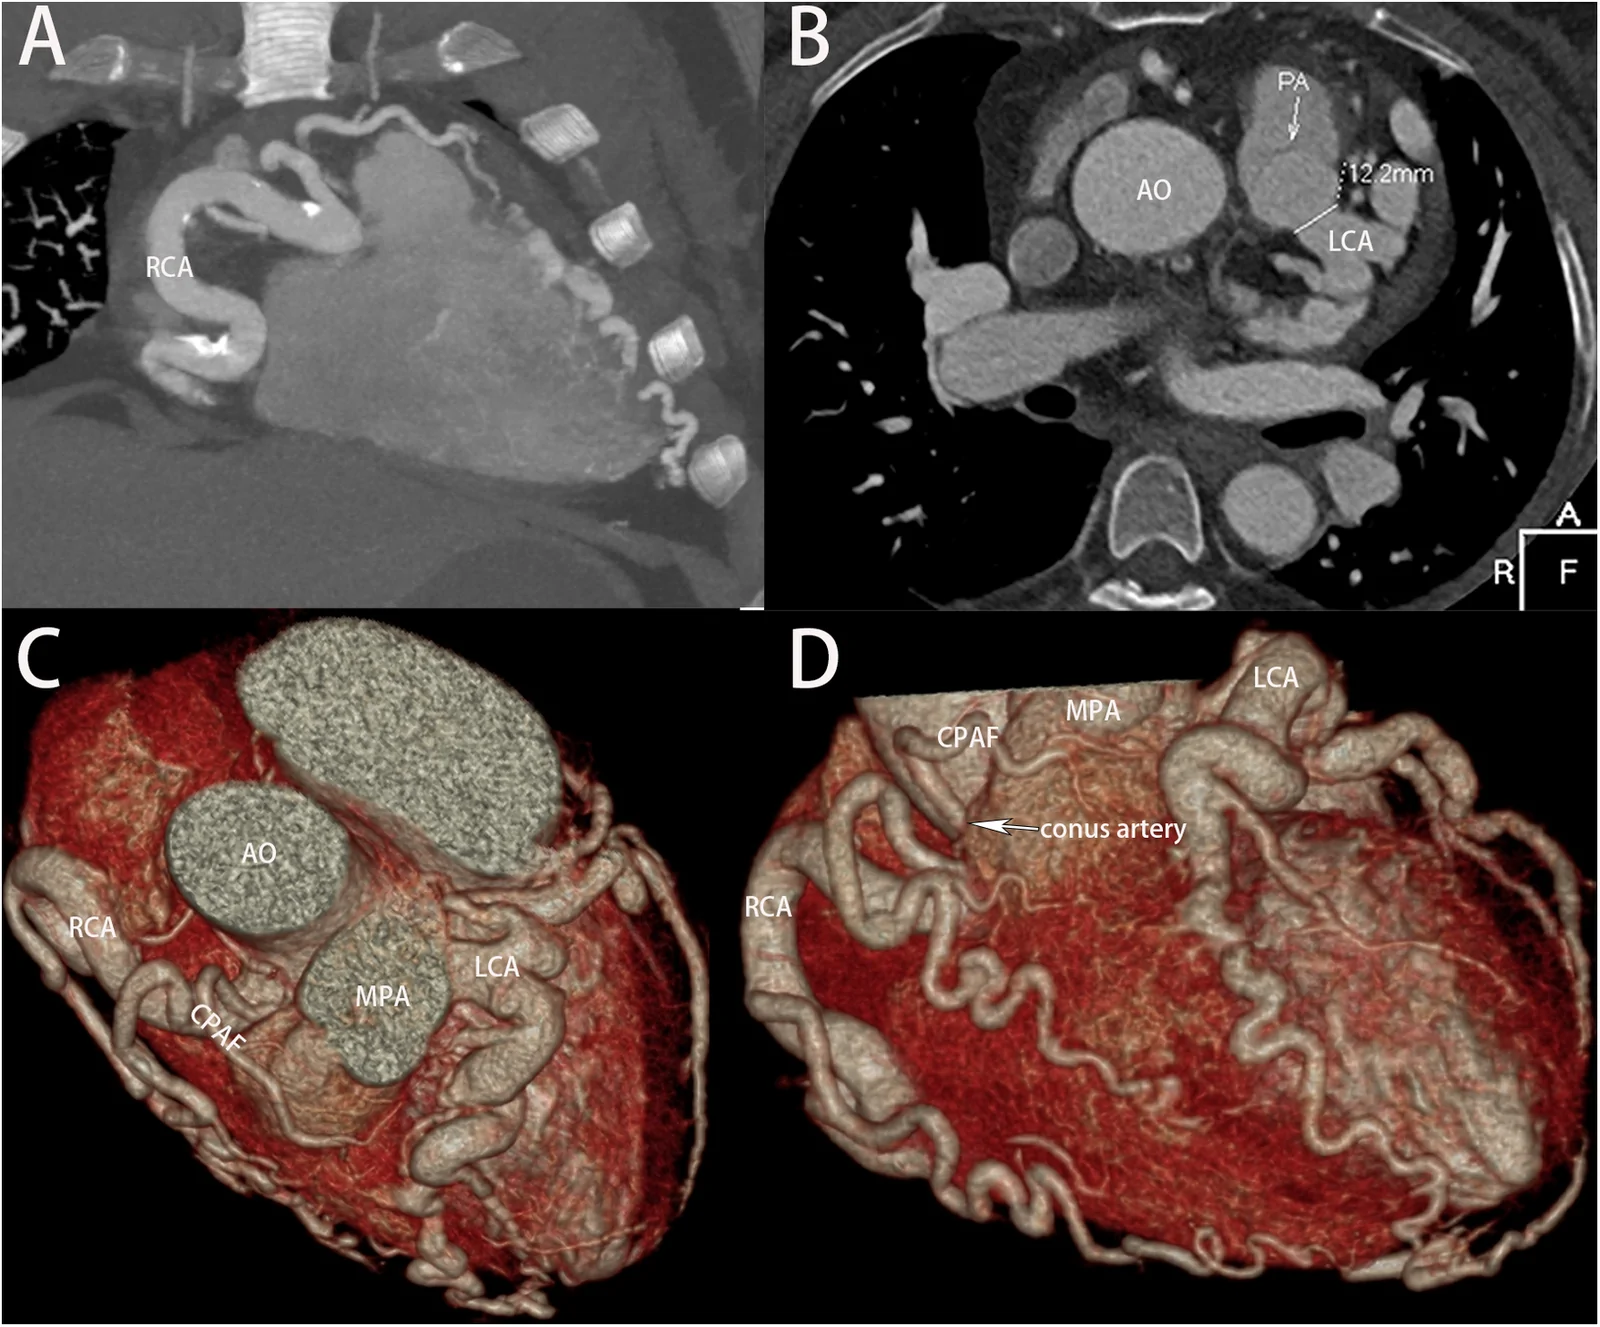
Coronary computed tomography angiogram showing right coronary artery calcification (A), an enlarged left coronary artery (B), an anomalous left coronary artery and a coronary-pulmonary artery fistula (C,D).
